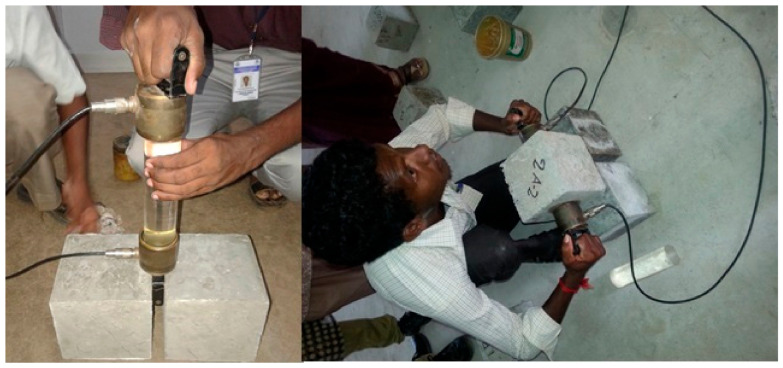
Test set up for ultrasonic pulse velocity test.
